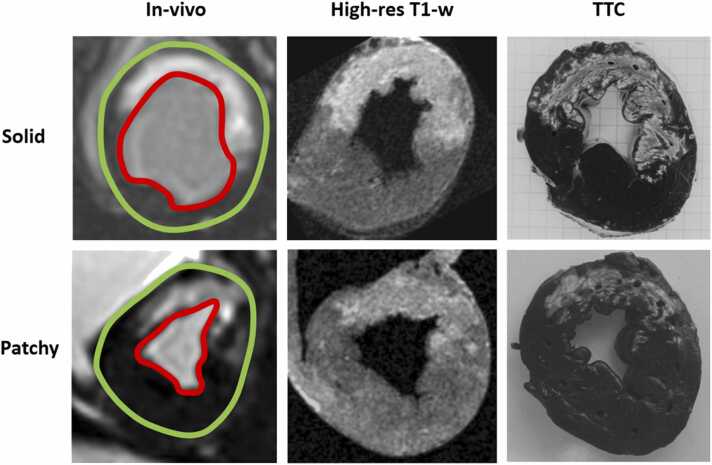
Comparison of solid and patchy infarctions. Top row shows a subject with solid infarction and the bottom row shows a patchy infarction. Left column shows in-vivo images where the endocardium is indicated in red and the epicardium in green. Middle column shows high-resolution T1w images used as reference standard and right column shows corresponding TTC images. Note that the patchy infarct shows a more insular infarction pattern, with areas of lower pixel intensity in both T1w and in-vivo LGE images. *TTC* triphenyltetrazolium chloride, *T1w* T1-weighted, *LGE* late gadolinium enhancement
